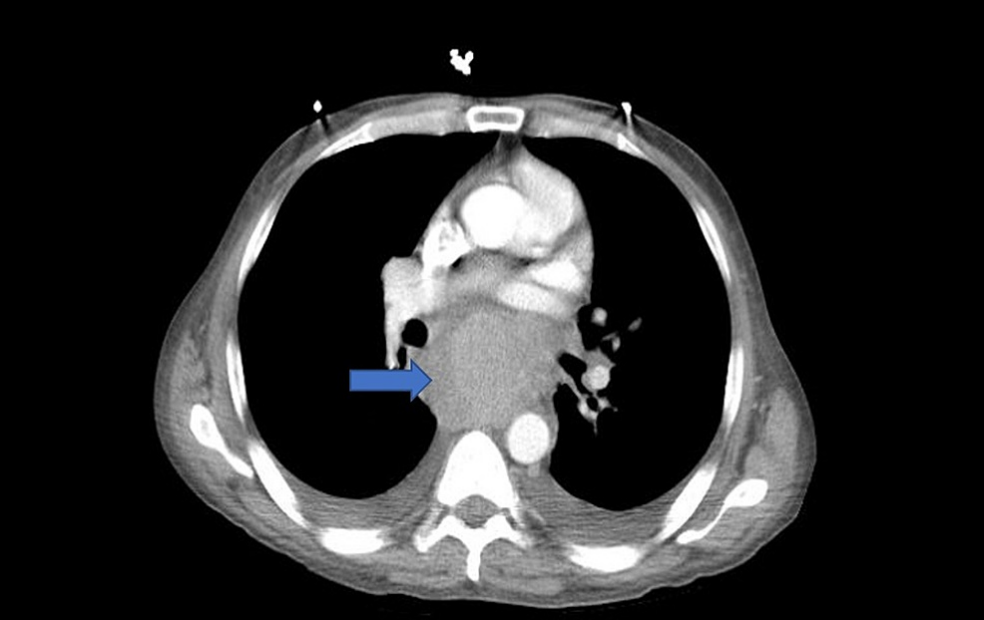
CT chest shows a subcarinal mass-like encirclement of the esophagus with splaying of the carina and mass-effect measuring 7.36 cm x 5.38 cm in its greatest dimensions (blue arrow)
